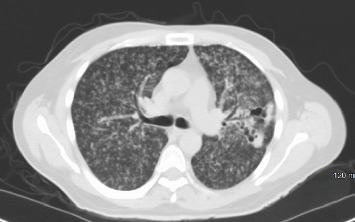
Thoracic CT scan without contrast demonstrating numerous, bilateral tiny nodular densities, most prominently in the upper lobes and confirmed a lingular cavitary lesion.
